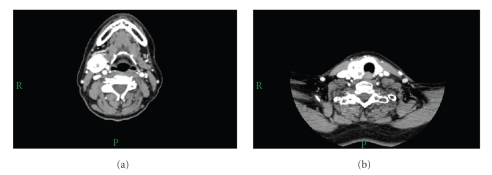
CT of neck with contrast. (a) Well-defined tumour mass in the right submandibular gland measuring 29 × 26 × 30 mm. It shows prominent vascularisation which would be unusual for benign lesion such as PA. There is some low-density area centrally in the tumour probably related to necrosis. (b) There is a large nodule in the thyroid on the right side measuring 26 × 23 × 30 mm showing similar intense enhancement as the submandibular lesion.
